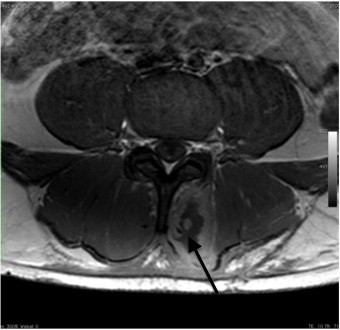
Magnetic resonance imaging (T1-weighted with gadolinium) showing cystic changes (arrow) in the multifidus muscle at 4 weeks after the operation.
